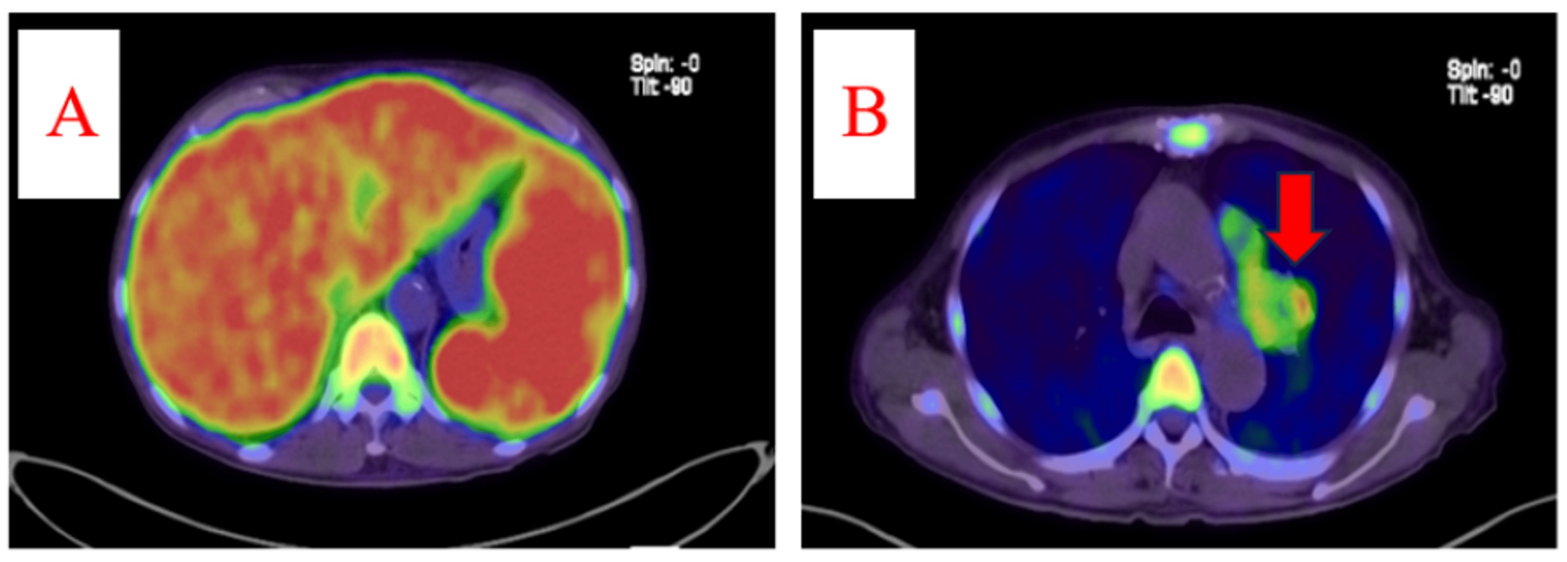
Positron emission tomography/computed tomography (PET/CT) showing (A) hepatosplenomegaly, with accumulation of contrast agent in the liver, spleen, and bone marrow, and (B) accumulation of contrast agent in a mass in the left upper lobe (red arrow)
